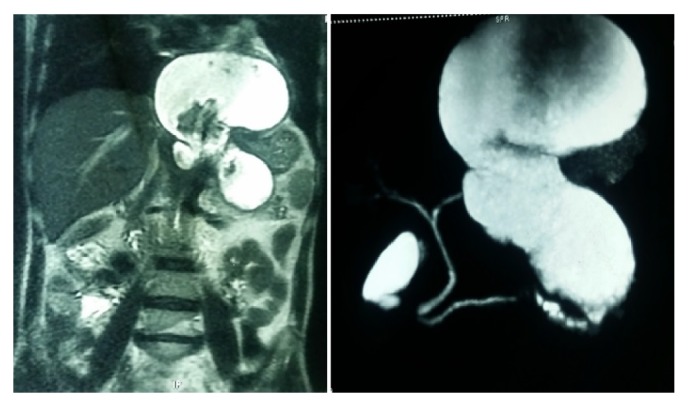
MRI abdomen showing pseudocyst involving tail of pancreas with mediastinal extension and MRCP showing ductal communication of the pseudocyst.
